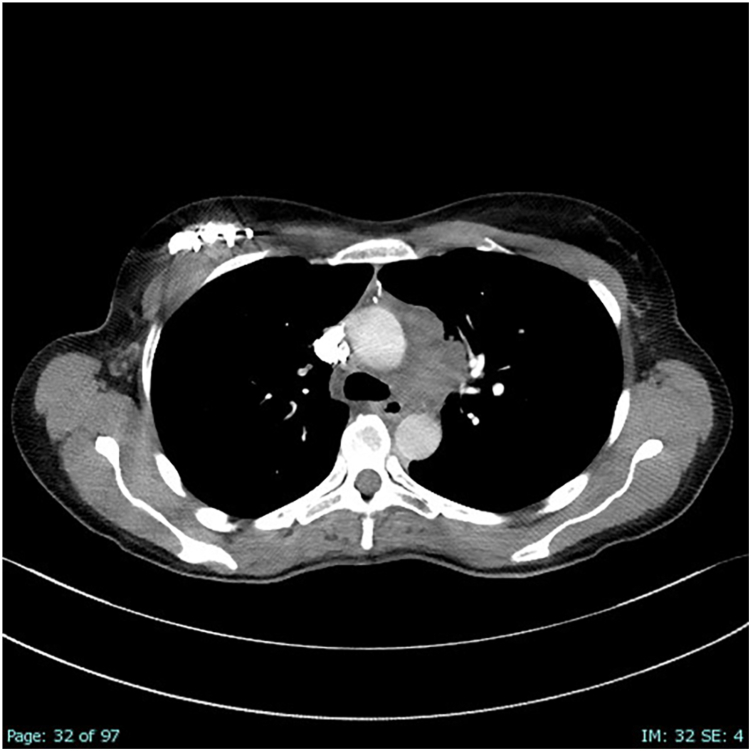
Initial computed tomography scan of the thorax showing the left-sided mediastinal mass.
